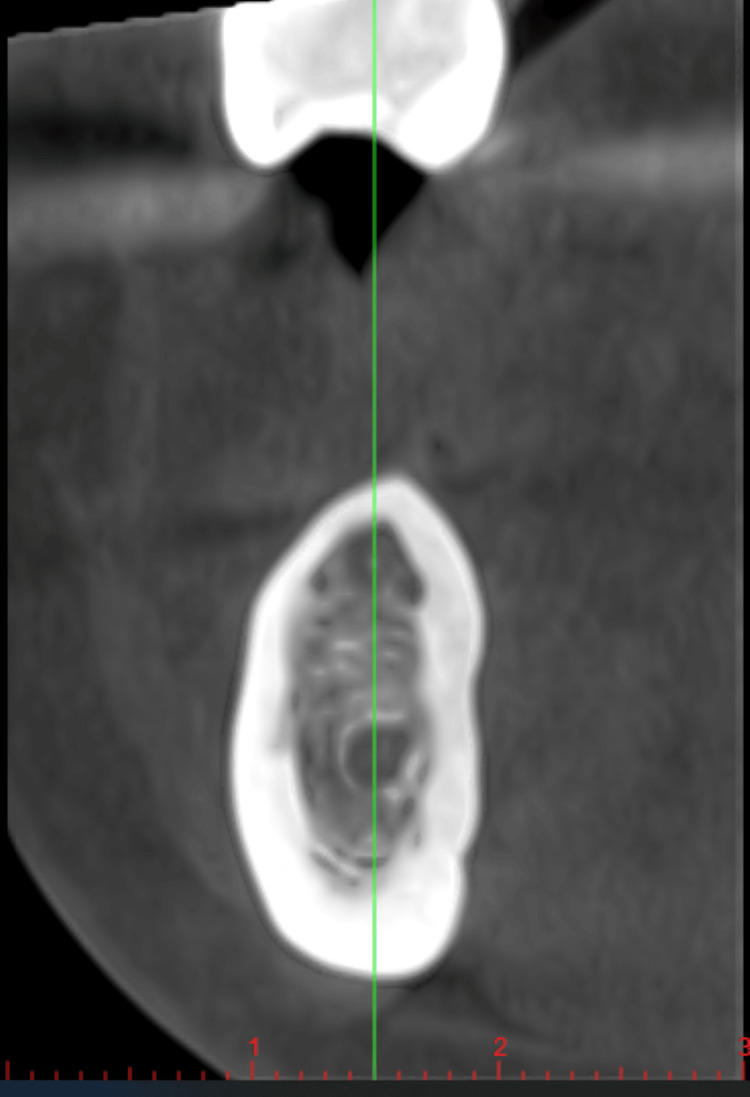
Cone beam computed tomography of edentulous area
